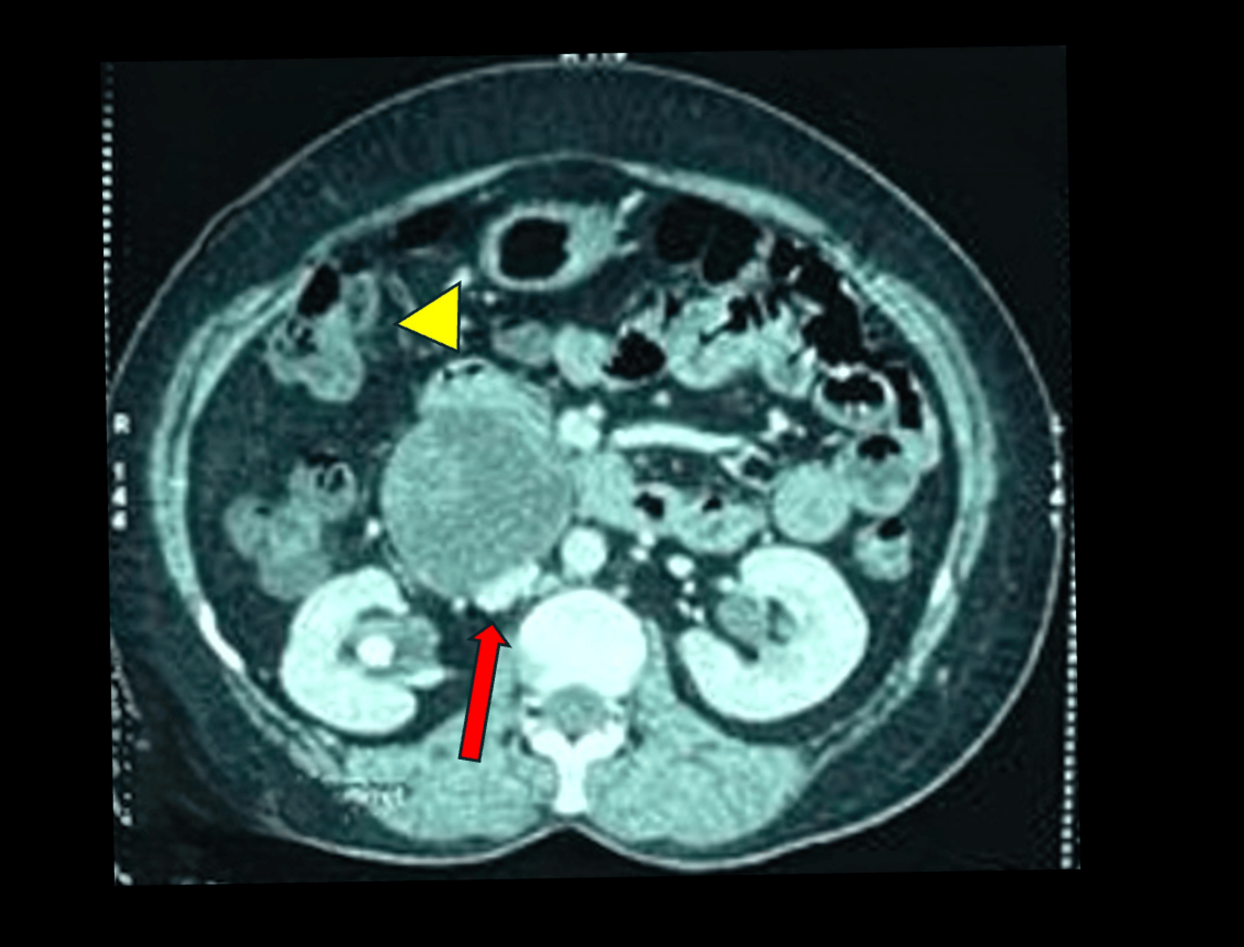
CT image showing the retroperitoneal heterogeneous cystic tumour and its relation to IVC and duodenum Red arrow - IVC, Yellow arrow head - duodenum IVC - inferior vena cava
